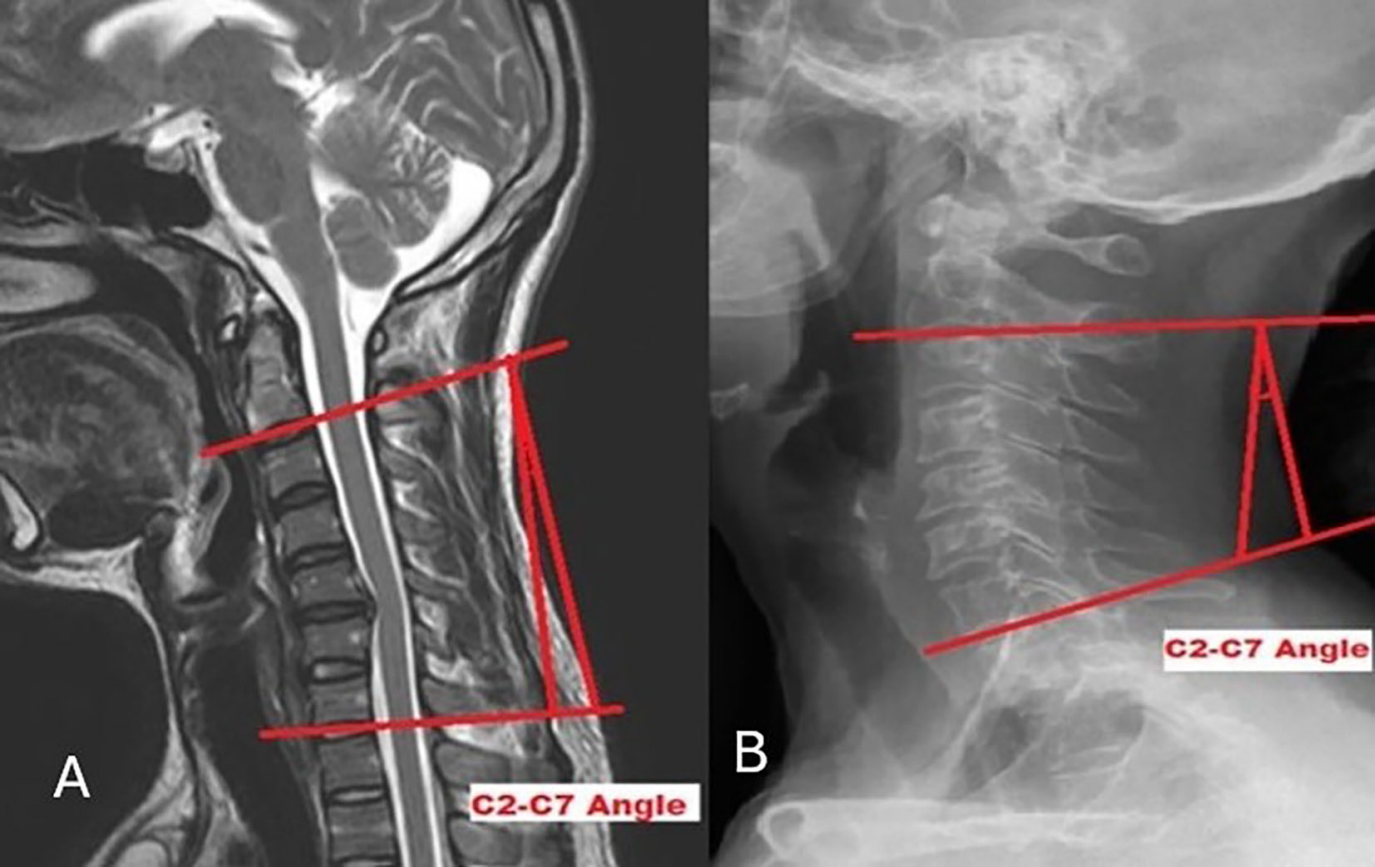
Measurement of C2-C7 angle on MRI (A) and radiograph (B).
